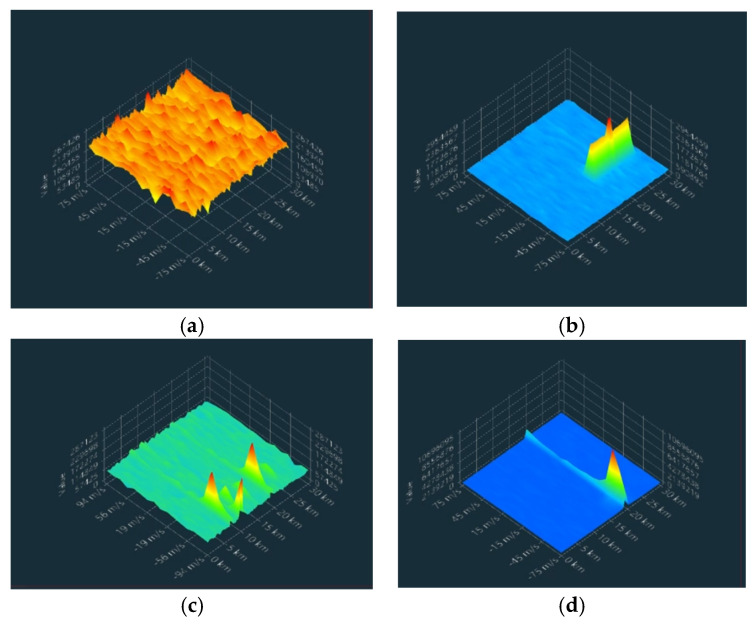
Measured range–Doppler maps obtained from the semi-physical platform under different jamming and anti-jamming conditions: (a) target echo under narrowband blocking jamming; (b) recovered target echo after applying the anti-NBJ strategy; (c) target echo and false-target echoes under repeater deception jamming; (d) recovered target echo after applying the anti-RDJ strategy.
